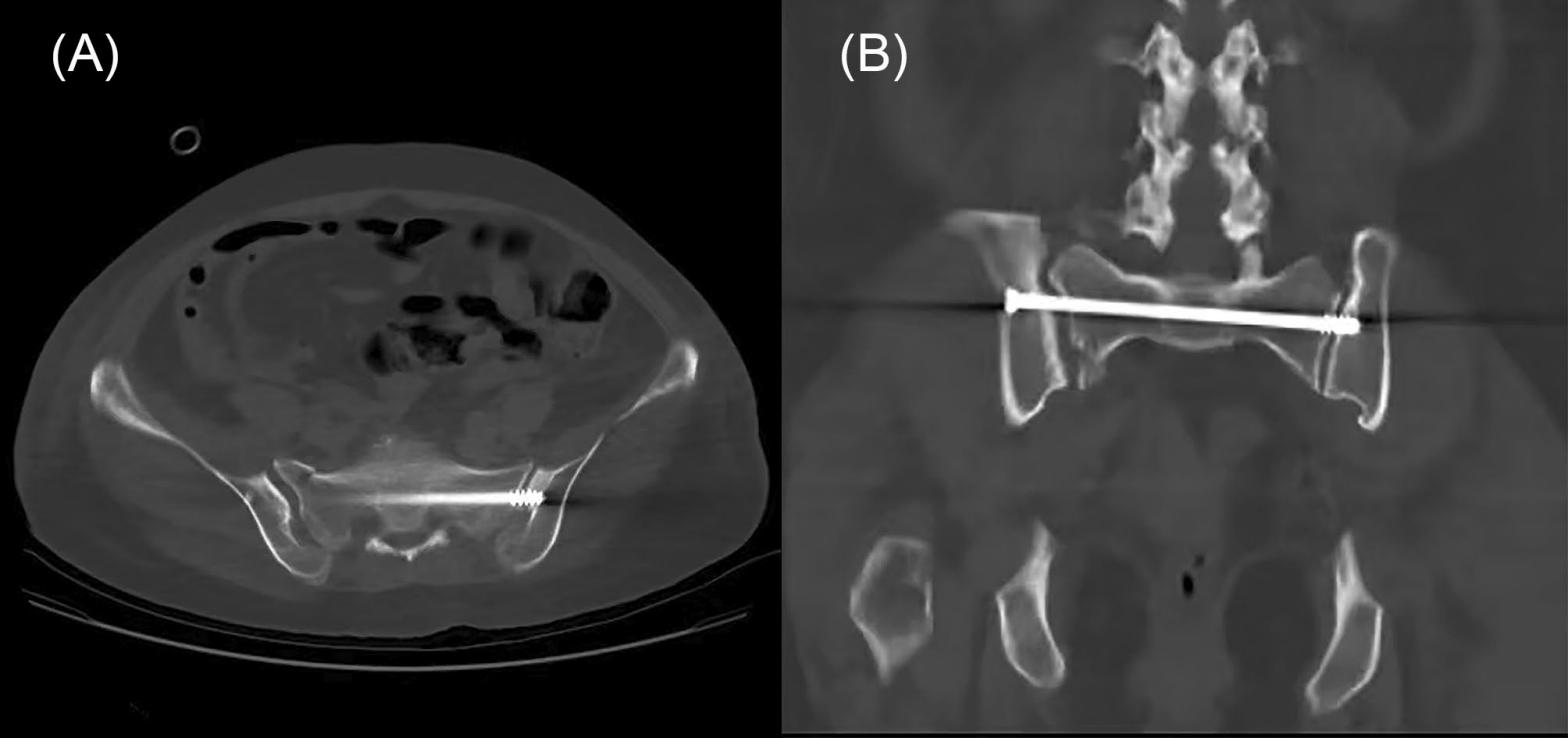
Imaging verification with rail-bound CT during operation. (A) Horizontal view. (B) Coronal view.
